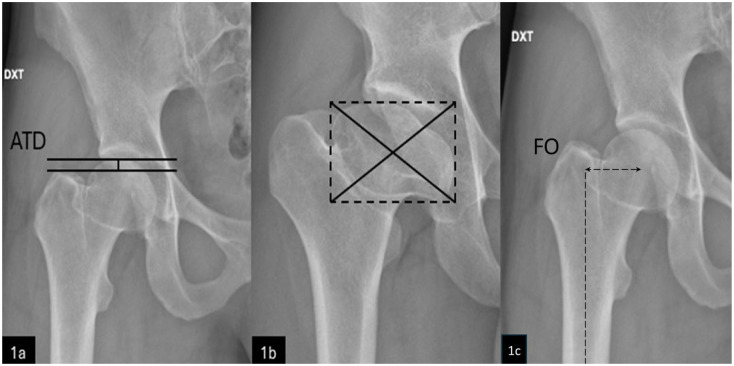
Radiographic assessment of 2 Perthes hips: (a) ATD: Distance between the 2 horizontal lines (solid). (b) Definition of centre of rotation: a rectangle is drawn outlining the medial, lateral, upper, and lower border of the femoral head (dotted lines), and diagonals (solid lines) mark the centre. (c) Femoral offset (FO): Distance from the centre of rotation of the femoral head to a line bisecting the long axis of the femur (dotted lines).
